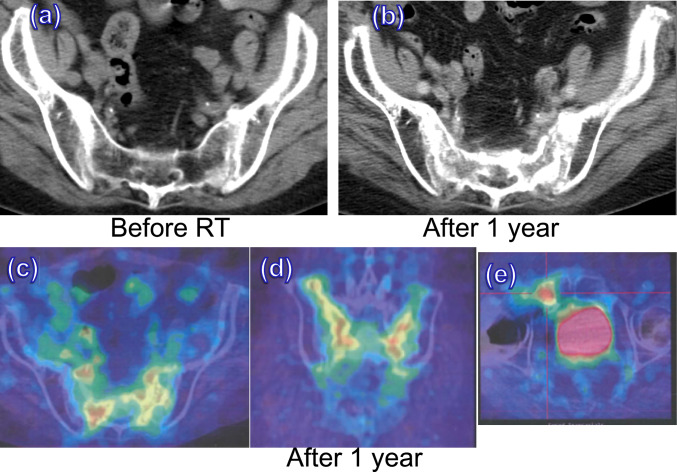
An 81-year-old woman who received RT alone for stage IIB cervical cancer with taking menatetrenone developed pain in lower back and buttocks without any particular incentive at 1 year after RT. She experienced difficulty walking, and was hospitalizes for detailed examination and treatment. (a) before RT (b) 1 year after RT: IF of sacroiliac joints (c) PET-CT 1 year after RT: uptake of bilateral sacroiloac joints increased; axial image (d) coronal image (e) IF of right pubic bone: uptake of right pubic bone increased
